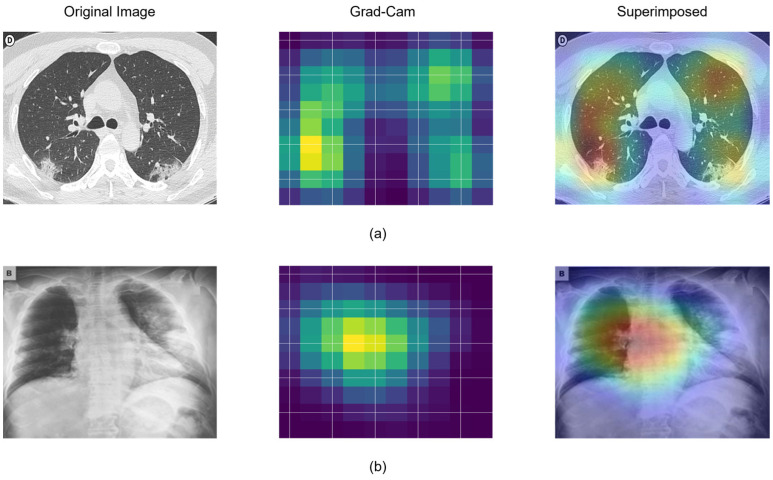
Grad-Cam visualization of the proposed SCovNet model for COVID-19 positive patient (a) CT scan and (b) CXR image.
